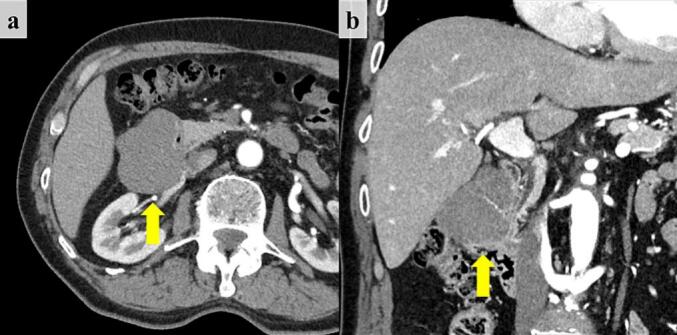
Abdominal computed tomography image. (a) Axial view: The 6.5 cm well-defined low-enhanced mass (arrow) is located in the second portion of the duodenum. (b) Coronal view.
